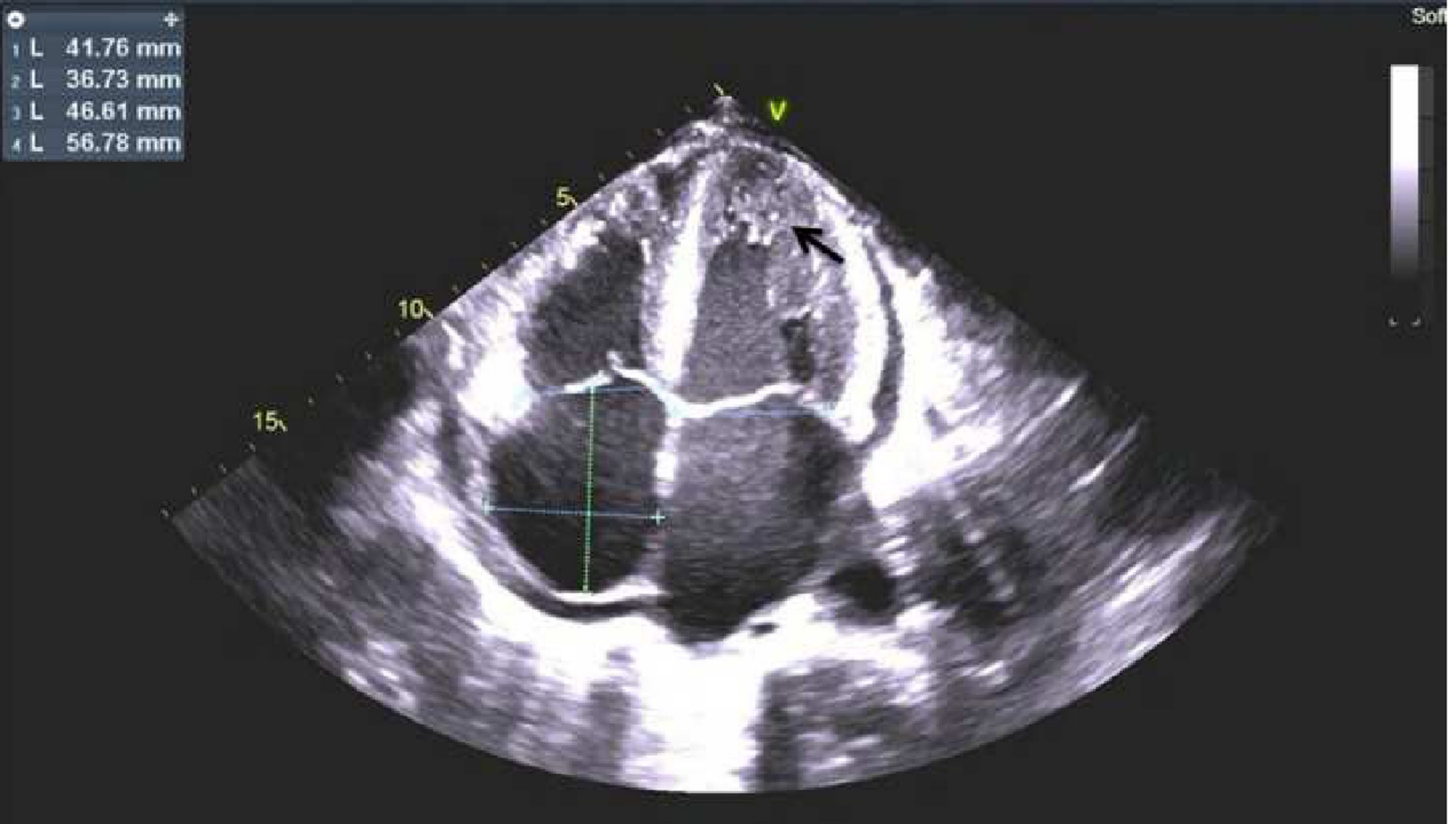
Echocardiography showing increased ventricular mass and granular sparkling pattern of myocardium (black arrows).
